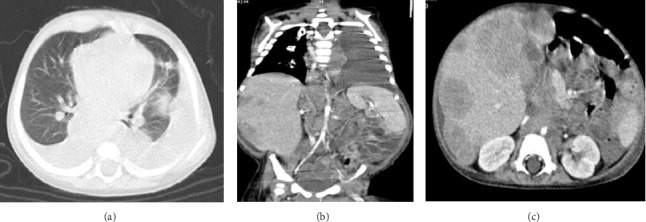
(a) Chest CT scan showed bilateral moderate pleural effusion. A large anterior mediastinal mass (45 × 30 mm) and left supraclavicular lymphadenopathies were observed. ((b) and (c)) abdominal-pelvic MRI revealed massive enlargement of the liver with numerous mass lesions, multiple conglomerated different-sized masses in retroperitoneum and lesser sac, associated with lymphadenopathies around aorta and its branches, superior mesenteric and renal arteries with extension to retrocrura and posterior mediastinum. The adrenals were spared.
